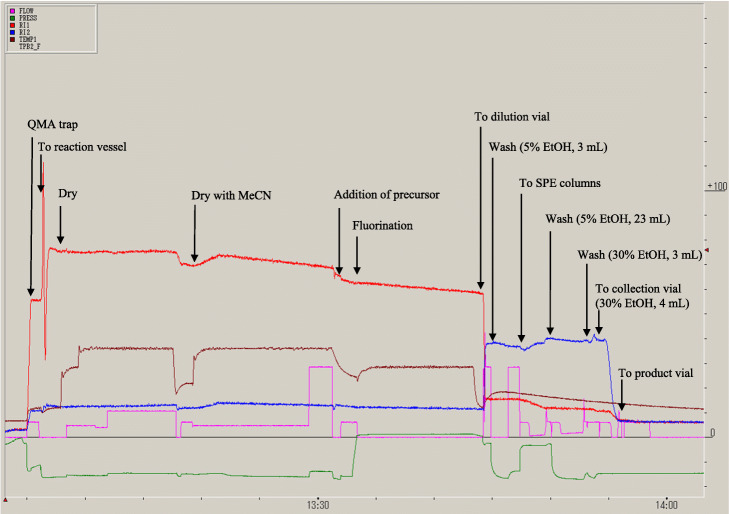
The typical trend graph of [^18^F]PSMA-1007 synthesis. Total synthesis time was 62 min on average. RI1 was installed via the reaction vessel (red line) and RI2 was installed via the dilution vial and solid phase extraction (SPE) columns (blue line)
